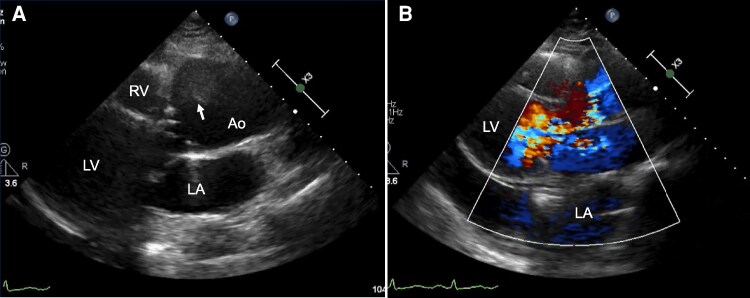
Transthoracic echocardiography findings. (*A*) Enlargement of sulcus of Valsalva, 50 mm, and movable linear structure suspected dissected intimal flap (arrow). (*B*) Severe aortic regurgitation was seen on the parasternal long-axis view. Ao, aorta; LA, left atrium; LV, left ventricle; RA, right atrium; RV, right ventricle.
